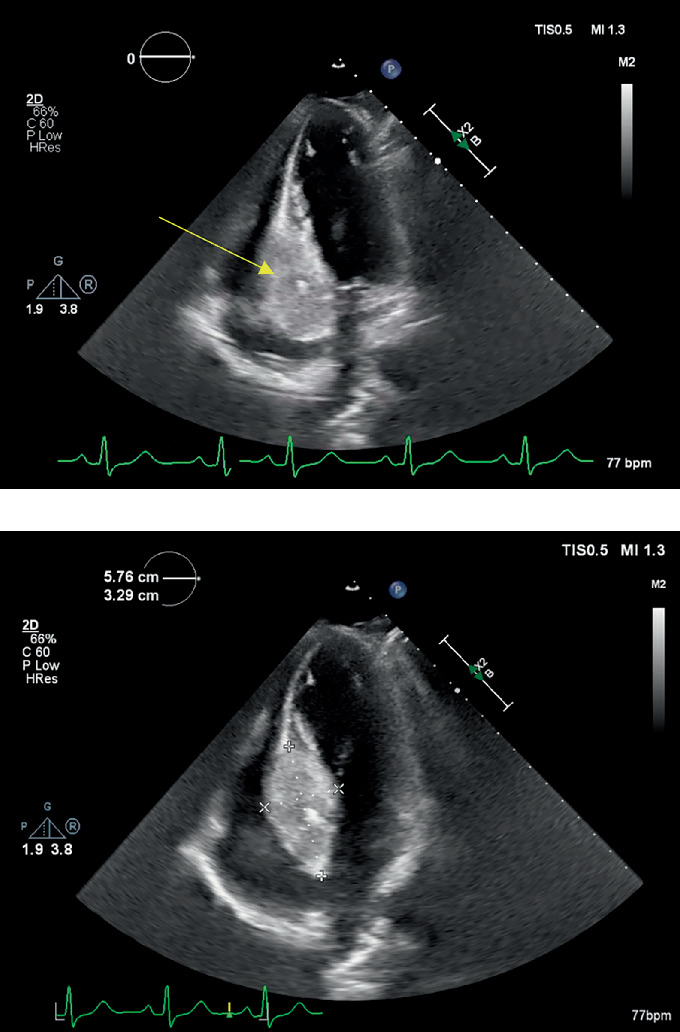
Transthoracic echocardiogram performed at an outside institution 2 years prior to presentation. (a) Apical 4-chamber view with arrow pointing to intracardiac mass located at the ventriculo-atrial septal junction, (b) measuring roughly 5.8 cm × 3.3 cm.
